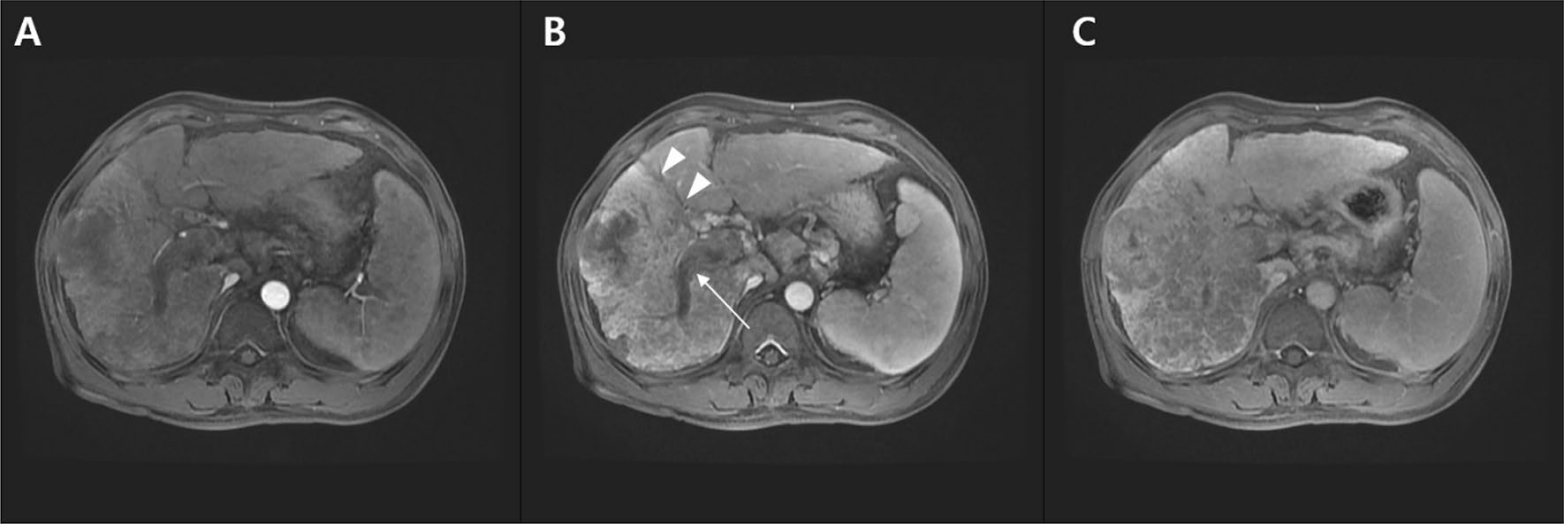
MRI image of a patient with cancer. Gadoxetic acid disodium-enhanced liver MRI images of a patient with HCC. (A) A huge mass replacing the right lobe of the liver in the arterial phase. (B) The mass (arrowhead) and portal vein thrombosis (arrow) are more prominent in the portal phase. (C) The mass shows washout in the venous phase.
